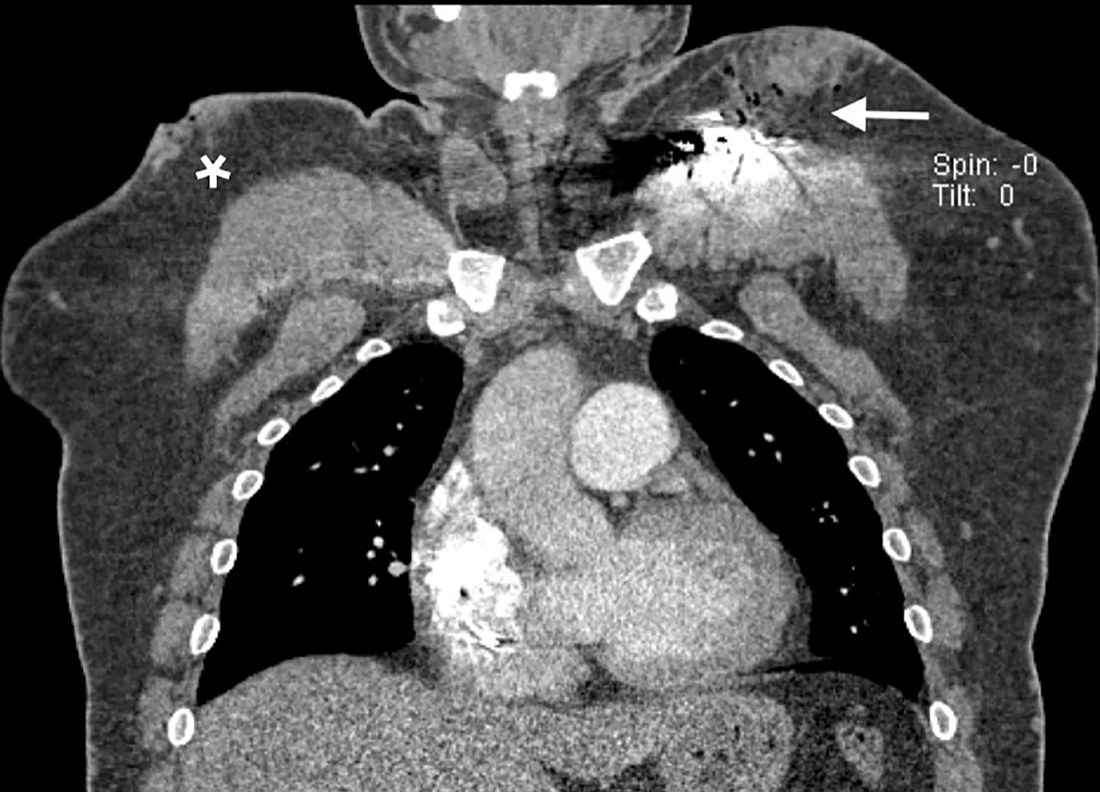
Representative computed tomography slice showing damaged automated implantable cardioverter defibrillator with surrounding subcutaneous air (arrow) as well as an additional gunshot wound to the right thorax (star).
